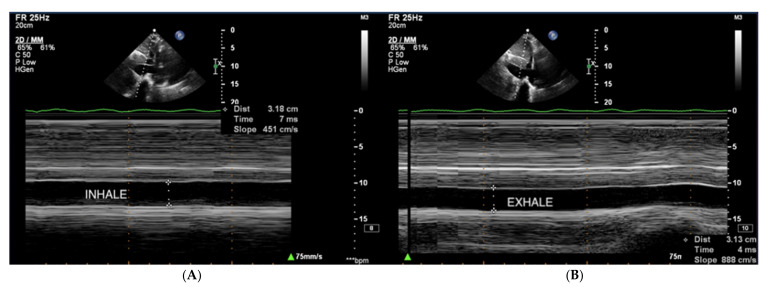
(A,B) IVC, no respiratory variability of IVC in the course of PAH. TTE, subcostal view. IVC: inferior vena cava; TTE: transthoracic echocardiography.
